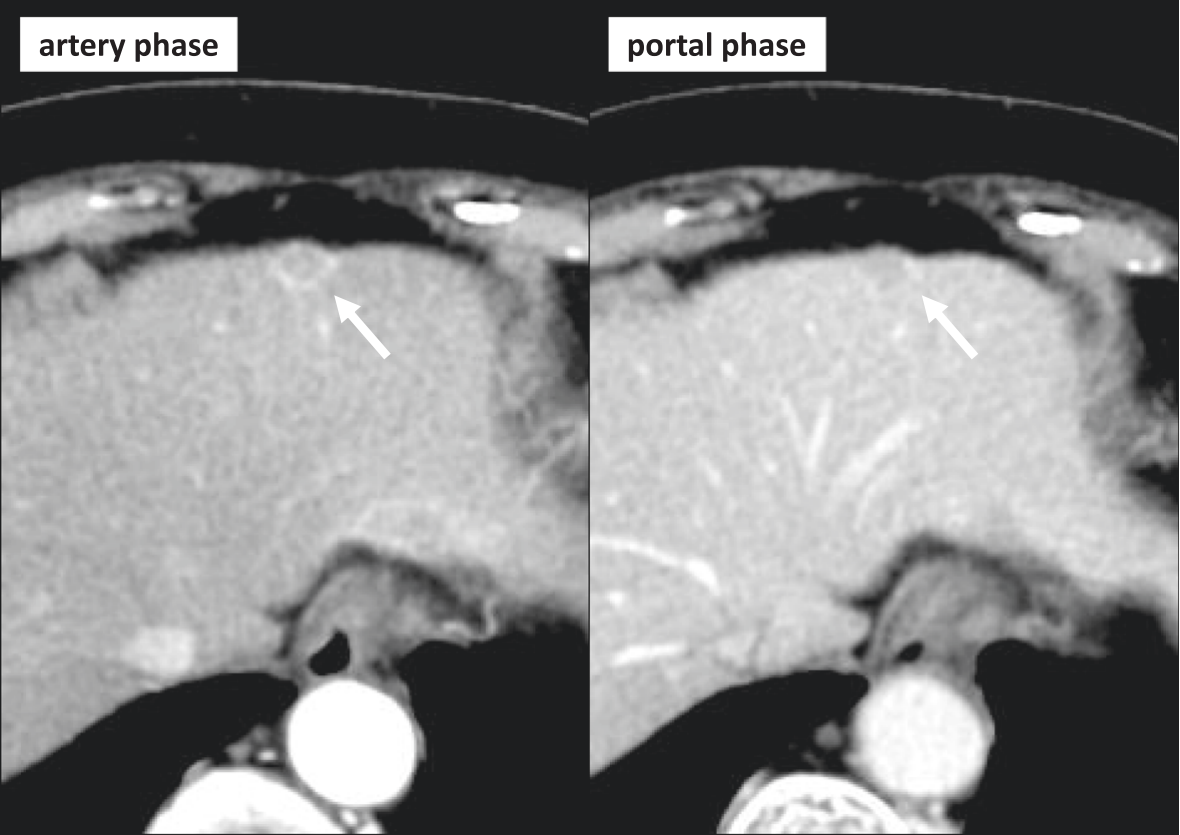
Contrast-enhanced computed tomography showing a nodule with ring enhancement in the arterial phase and subsequent washout in the portal phase in segments 2, 3 (*arrow*), 4, and 8 of the liver
